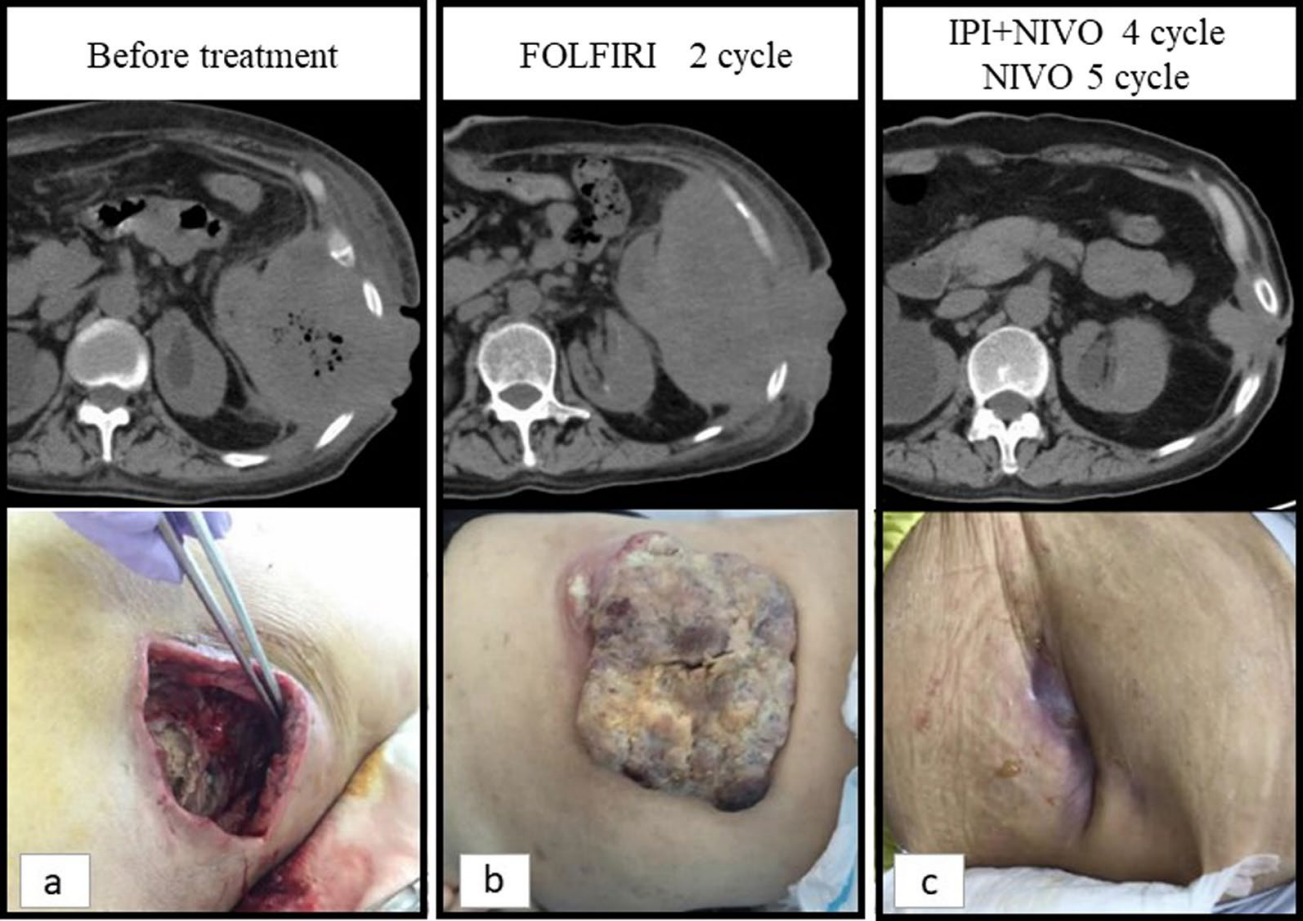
Computed tomography and pictures of the tumor according to the course of anticancer therapy. a Before treatment. b After two cycles of FOLFIRI therapy. c After four cycles of nivolumab and ipilimumab combination therapy and five cycles of nivolumab monotherapy
